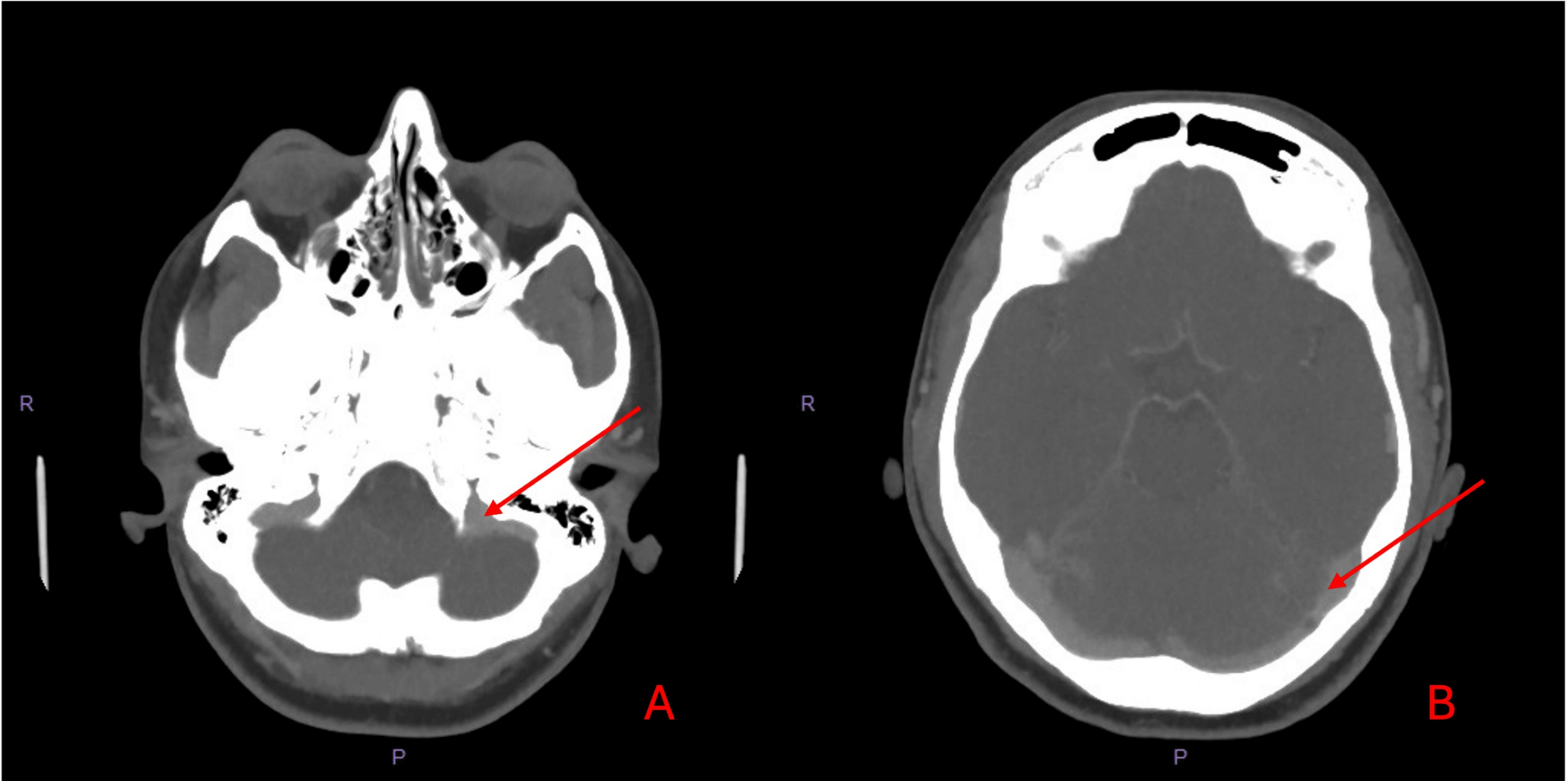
CT venogram ruling out a thrombus in the sigmoid and transverse sinuses A: CT venogram showing no occlusion in the left sigmoid sinus (arrow). B: CT venogram showing no occlusion in the left transverse sinus (arrow).
